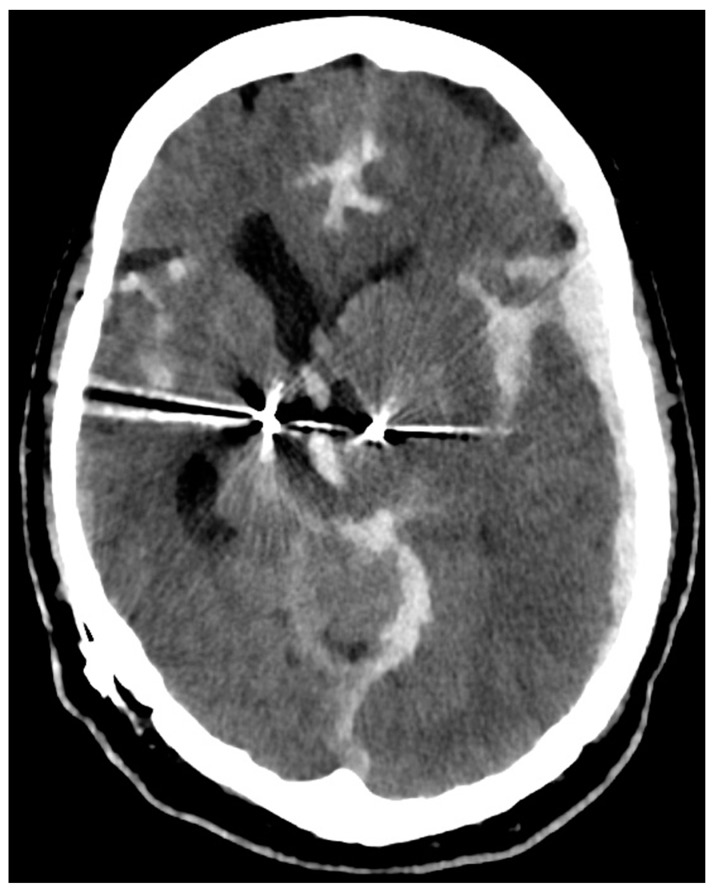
Initial head CT scan shows the predominantly left-sided subarachnoid hemorrhage with intra-ventricular hemorrhage, subdural hematoma, and midline-shift. The DBS stimulation leads are displaced to the contralateral side.
